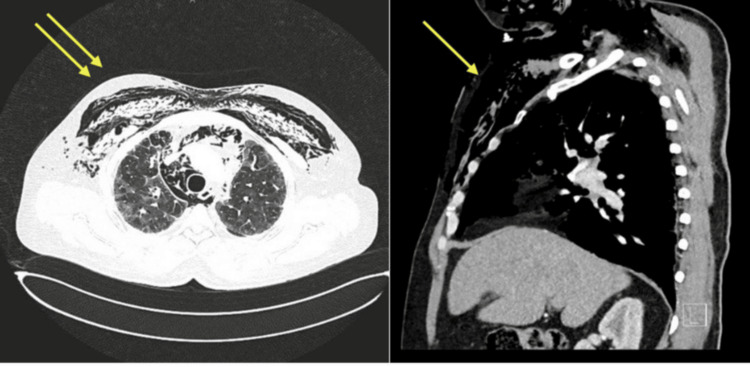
(A, B) CT chest showing extensive subcutaneous emphysema (yellow arrows) tracking through the chest wall and soft tissues (axial and sagittal views).
